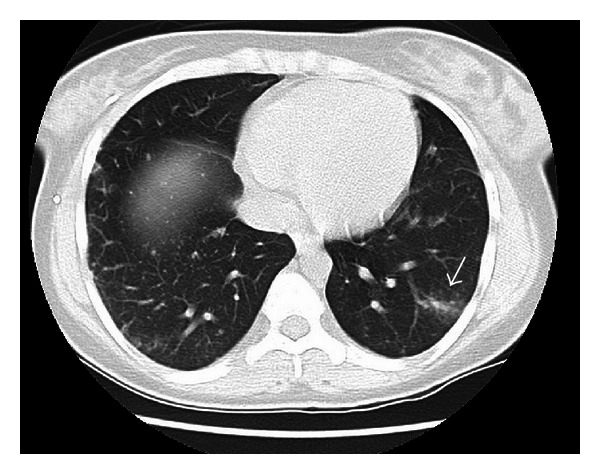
Compute tomography (CT) of chest showing wedge-shaped ground glass opacity in the left lower lobe, with surrounding centrilobular nodules (arrow). Additional 1-2 mm centrilobular nodules noted in the right lower lobe.
